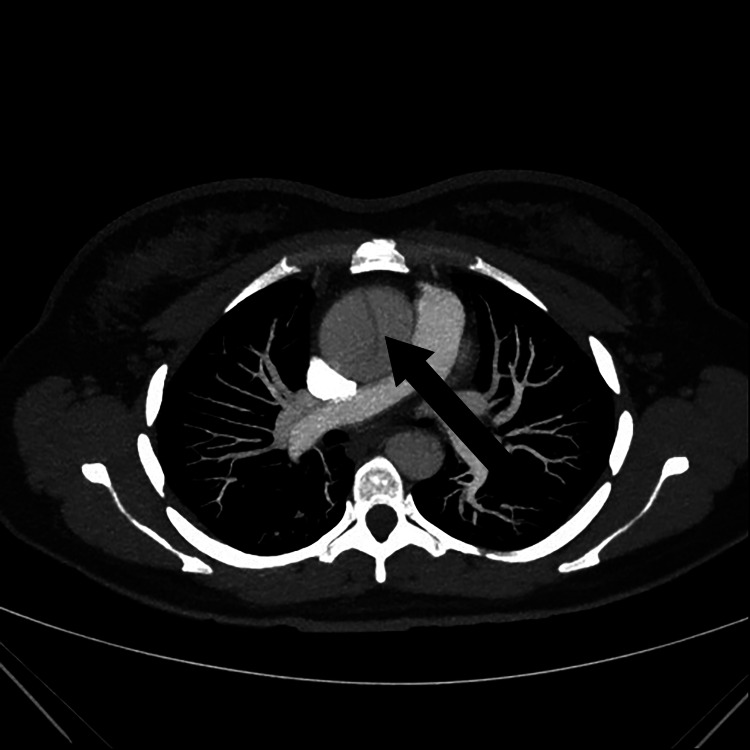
CT axial image of the ascending aortic aneurysm dissection (black arrow) CT: computed tomography
